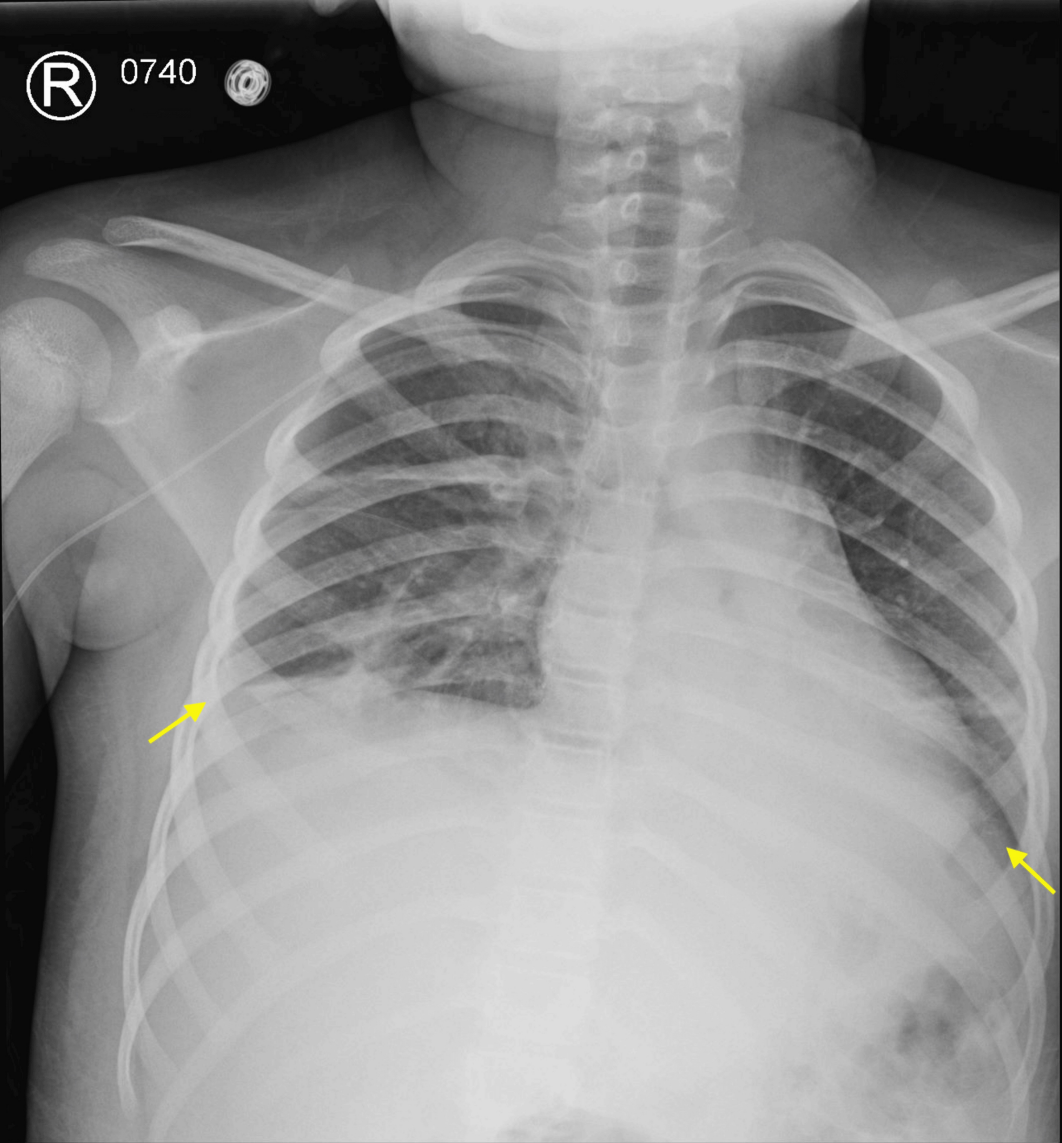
Chest x-ray demonstrating bilateral pleural effusions
